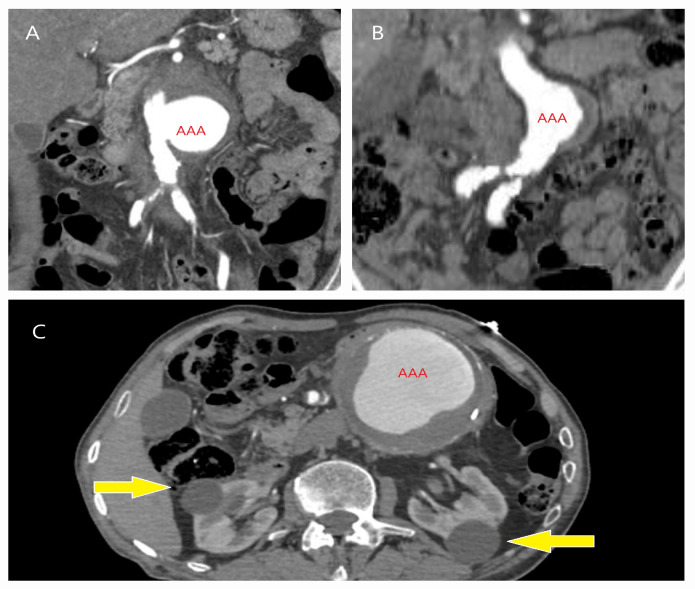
CT images of abdominal aortic aneurysm (AAA) cases incidentally detected during evaluations for unrelated clinical indications. (A) A saccular aneurysm was identified in a 60-year-old male during an emergency CT scan after a traffic accident. (B) An asymptomatic aneurysm was detected in a 68-year-old hypertensive male with a heavy smoking history (≥2 packs/day) during a prostate cancer evaluation. (C) An aneurysm was discovered in a 58-year-old female during a CT scan assessing the vena cava and iliac veins after developing deep vein thrombosis in the left lower extremity while being monitored for cystic kidney disease (yellow arrows) and atrophic kidney.
